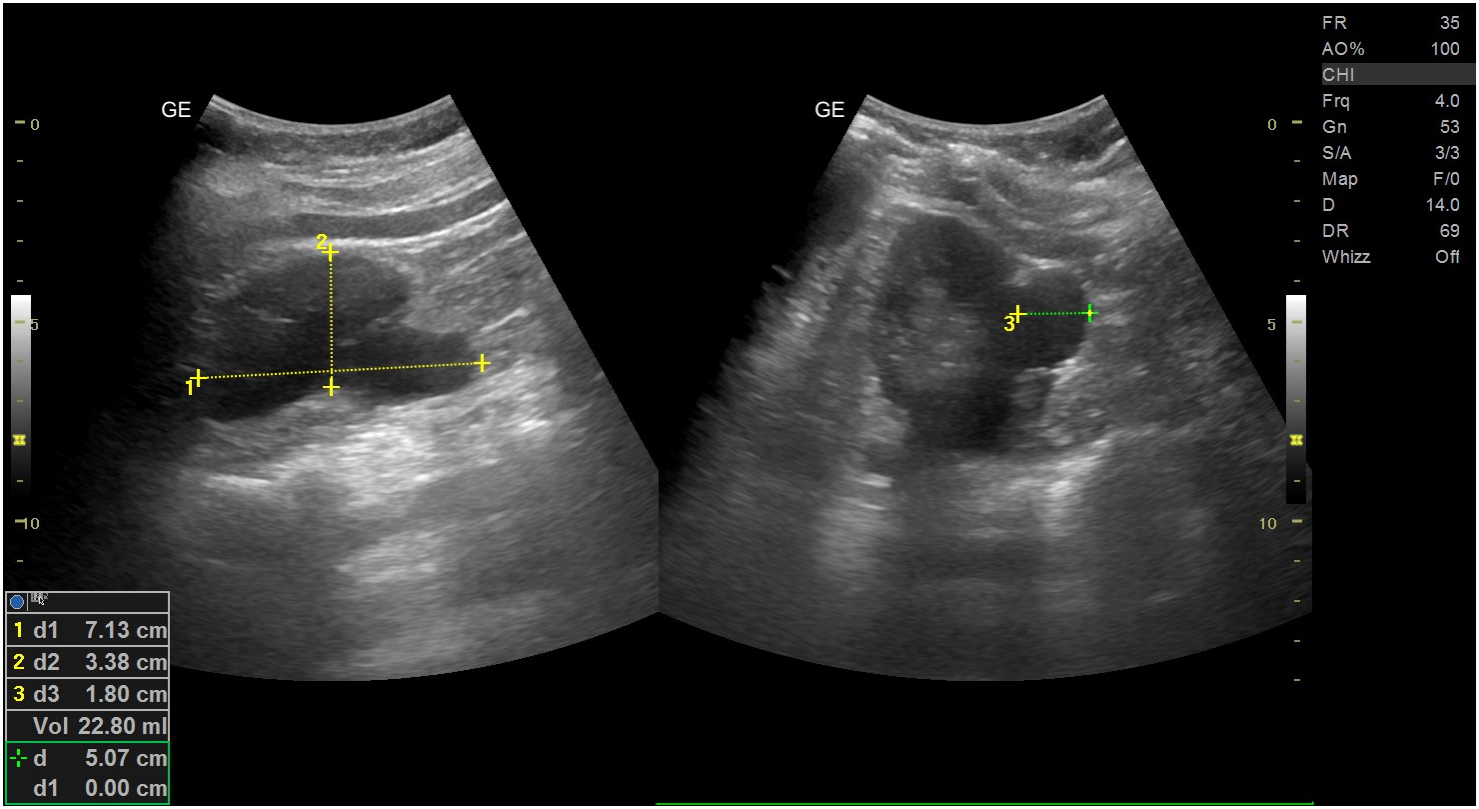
Ultrasonography representing left perinephric collection (abscess) with linear echogenic content.
